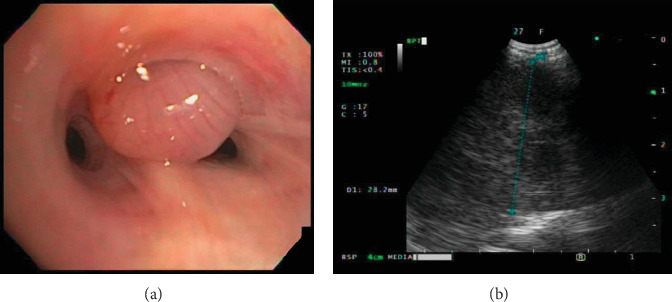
(a) Endobronchial smooth polypoid lesion arising from the right upper lobe apical segment. (b) Endobronchial ultrasound image of the right paratracheal heterogeneous mass.
